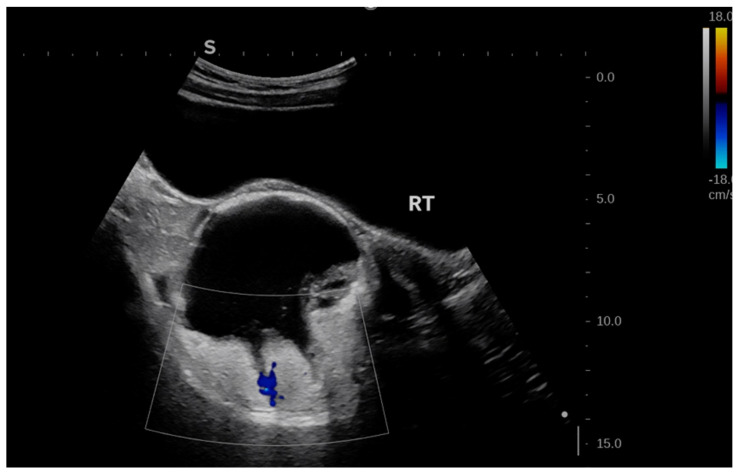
Colour Doppler flow imaging shows the vascular signals within the hyperechoic areas. No distinct blood flow signals were observed within the anechoic areas.
